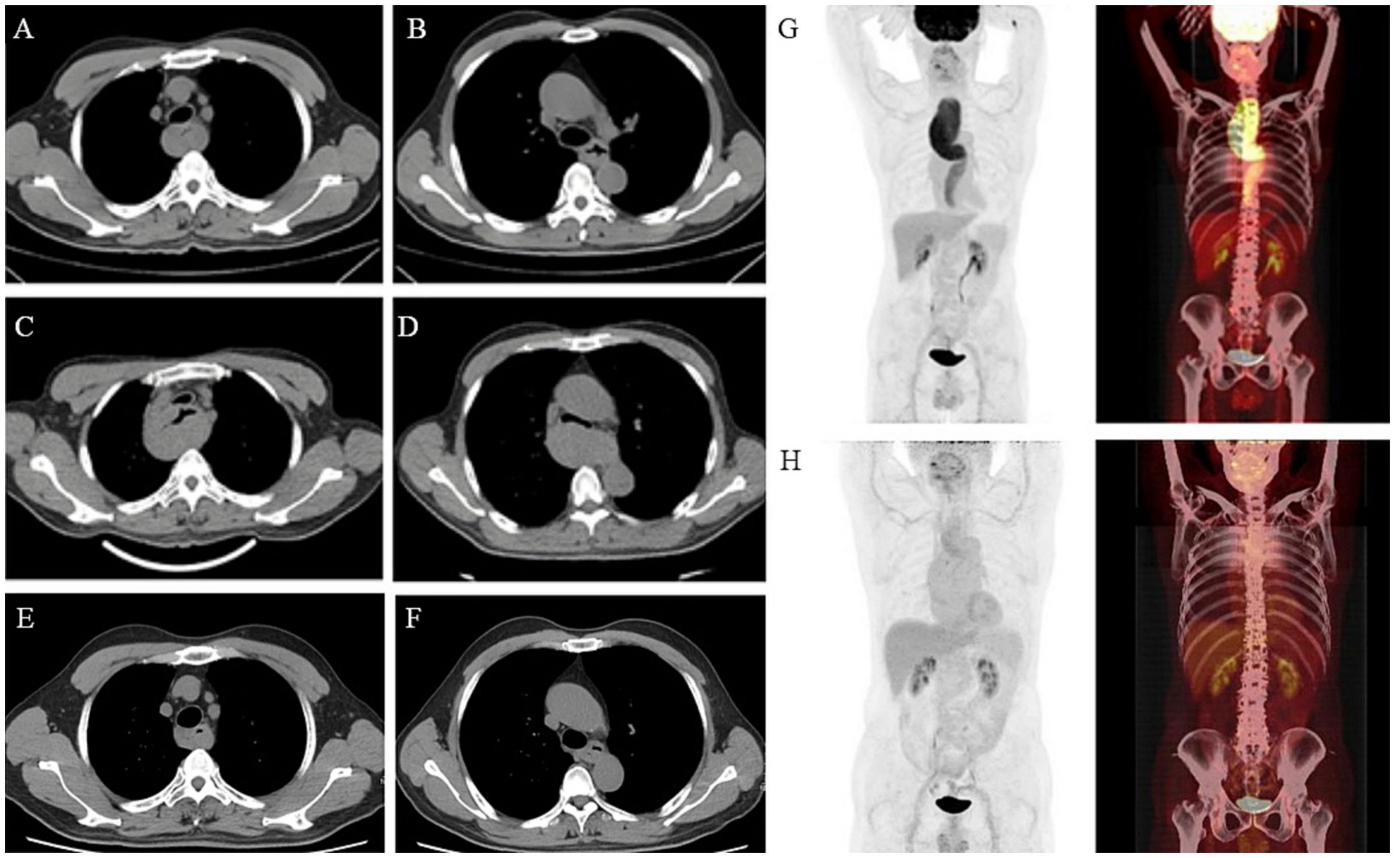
Findings of chest CT and PET-CT in 2022, 2024, and 2025. (A,B) Chest CT in 2022: multisegmental thickening of the esophageal wall and slight local compression of the trachea. (C,D) Chest CT taken in 2024 revealed that the esophageal mucosa thickened significantly with severe local pressure in the trachea and that there was no significant lymph node enlargement in the mediastinum. (E,F) Chest CT in 2025: significant improvement in esophageal mucosal thickening compared to previous scans, with reduced compression on the trachea. (G) PET-CT in 2024: revealed irregular thickening of the entire esophageal wall and an uneven increase in radioactivity uptake. (H) PET-CT in 2025: reduced esophageal wall thickening compared to previous scans, suggesting partial remission.
